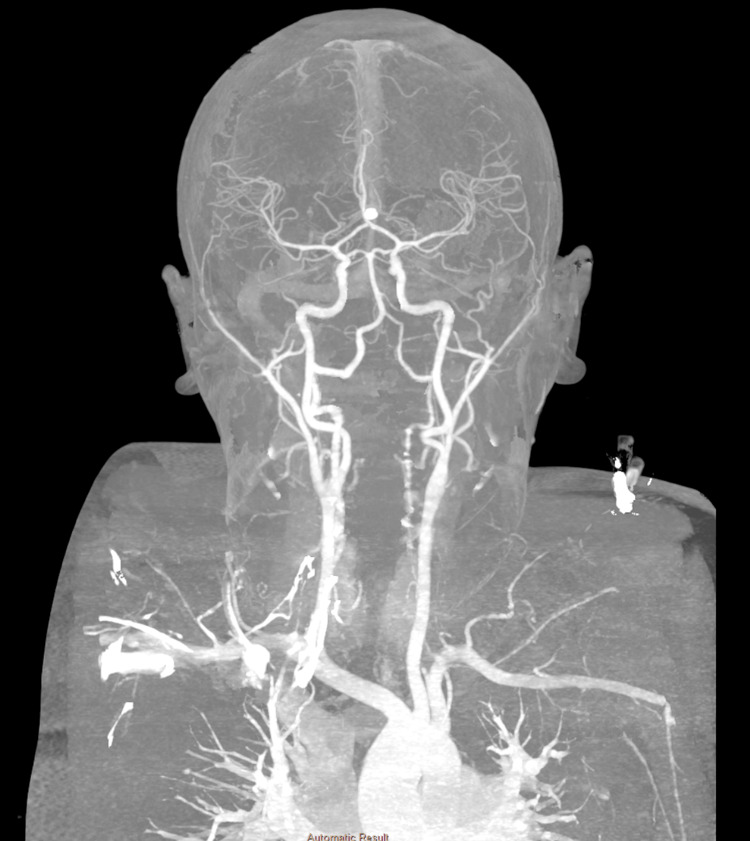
CT angiography reconstruction from the stroke code protocol showing no large vessel occlusion
